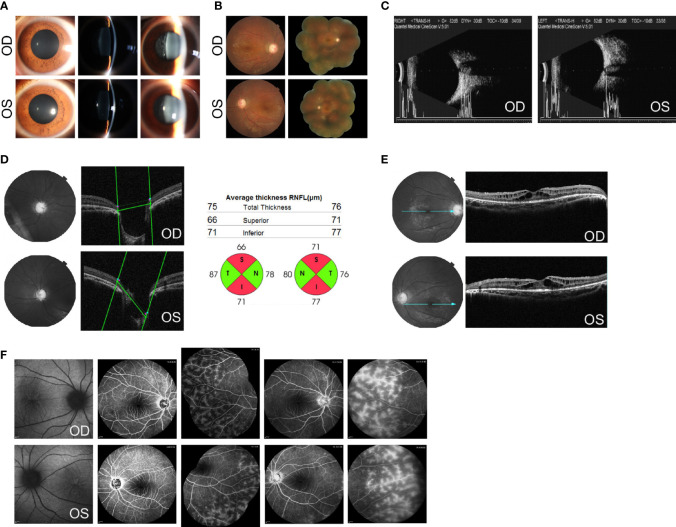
Ocular findings at the initial examination. (A) Slit-lamp aspects of the ocular anterior segment show moderate mydriasis, shallow anterior chamber, lucent lens, and vitreous opacities with cells in both eyes. (B) Fundus photographs show a C/D of 0.8 and dull macular reflex in the posterior pole, without pigmentary changes in both eyes. (C) B-mode ultrasound scanning shows a short axial length (20.0 mm for the right and 20.2 mm for the left) with optic disk excavation in both eyes. (D) Optical coherence tomography (OCT) shows an enlarged and depressed optic disk with RNFL thickness in both eyes. (E) OCT shows macular retinoschisis in the inner and outer nuclear layers, with the disruption of the ellipsoid zone and external limiting membrane within the fovea. (F) Fundus autofluorescence (AF) shows foveal hyperfluorescent specks in the right eye and streaks of radial hyper-AF originating from the central fovea in the left eye. The early and late phases of FA show spoke-wheel-like dye accumulation without leakage within the fovea and peripheral retinal telangiectasia with mild leakage in both eyes. OCT images of the optic disk and values of RNFL thickness were acquired using Topcon 3D OCT-2000 (Topcon Corporation, Tokyo, Japan). OCT images of the macula were acquired using Heidelberg SPECTRALIS OCT (Heidelberg Engineering, Heidelberg, Germany). AF images were acquired using Heidelberg SPECTRALIS HRA (Heidelberg Engineering, Heidelberg, Germany).
